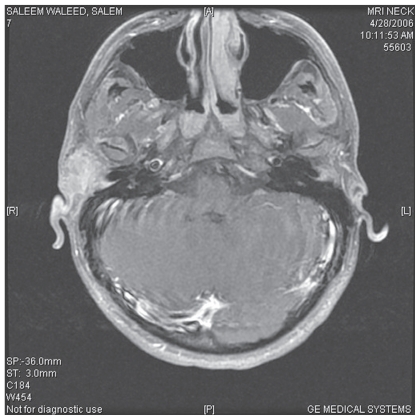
MRI study showed a 3.25 × 2.25 × 4.5 cm mass extending to the Masseter muscle and petrous bone medially, the cartilaginous part of the external auditory meatus posteriorly and the upper border of the right parotid gland inferiorly.
